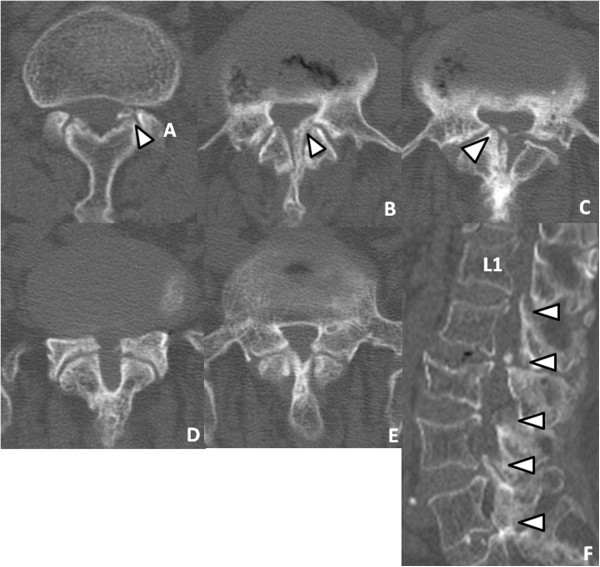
Preoperative computed tomography. (A) through (E) Preoperative computed tomographic scans show congenital spinal stenosis from L1 to L5, respectively. Axial images show ossification of the ligamentum flavum (OLF) at L1/2 (A), L2/3 (B) and L3/4 (C) (arrowheads). (F) Sagittal scan shows OLF at L1/2 (A) to L3/4 (C) and spinal stenosis with thickened lamina and narrowing of the lateral recess at L4/5 and L5/S (arrowheads).
